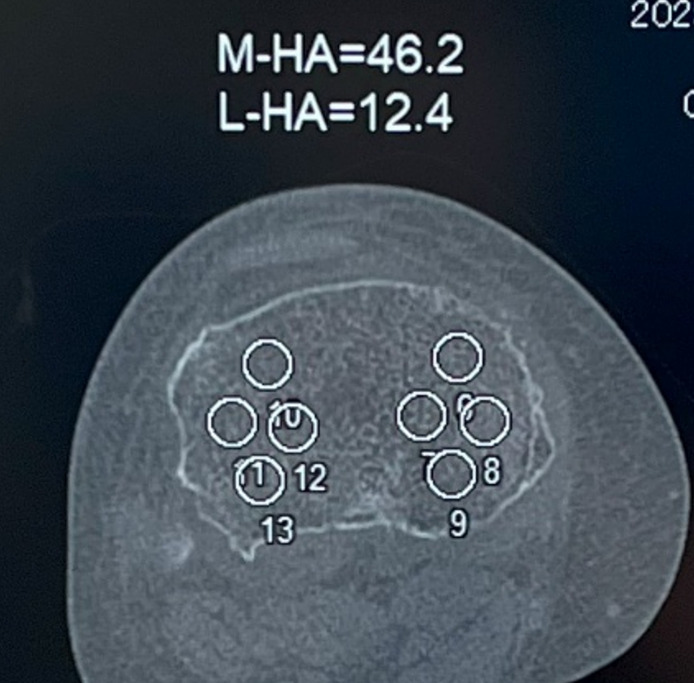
Location of CT measurements for medial and lateral tibial bone mineral density. HA, hydroxyapatite; L, lateral; M, medial.
